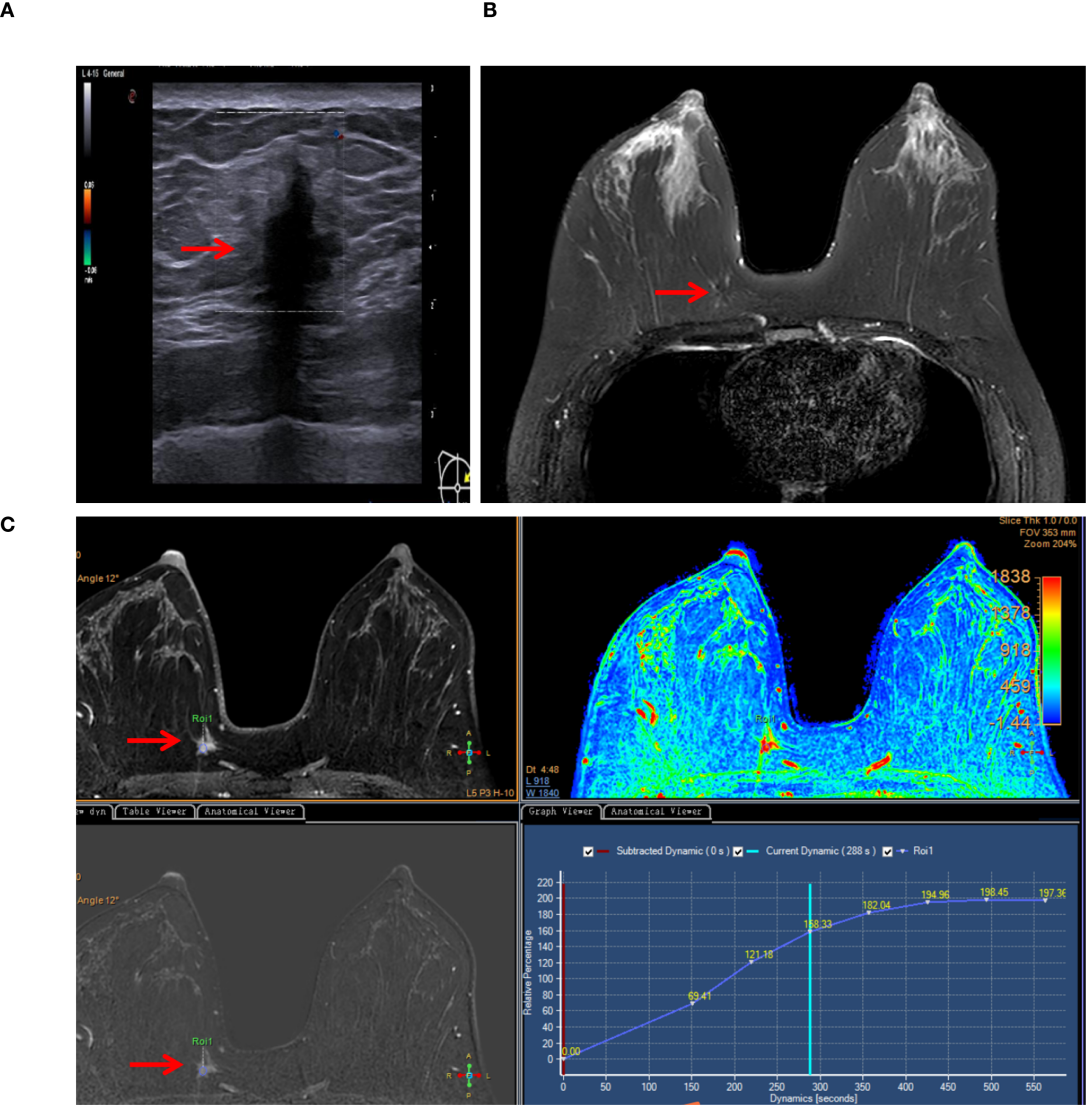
Typical imaging findings (lesion marked by arrows). (A) Color Doppler ultrasonography detected a noncapsular mass in the upper inner quadrant of the right breast (15×15×14 mm). (B) Breast MRI revealed a mass in the right breast (15×13×8 mm), exhibiting slightly high signal intensity on T2-weighted imaging (T2WI). (C) MRI dynamic contrast enhancement, silhouette image, Apparent diffusion coefficient (ADC) mapping (pseudo-color image), dynamic contrast enhancement and the time-intensity curve (TIC).
